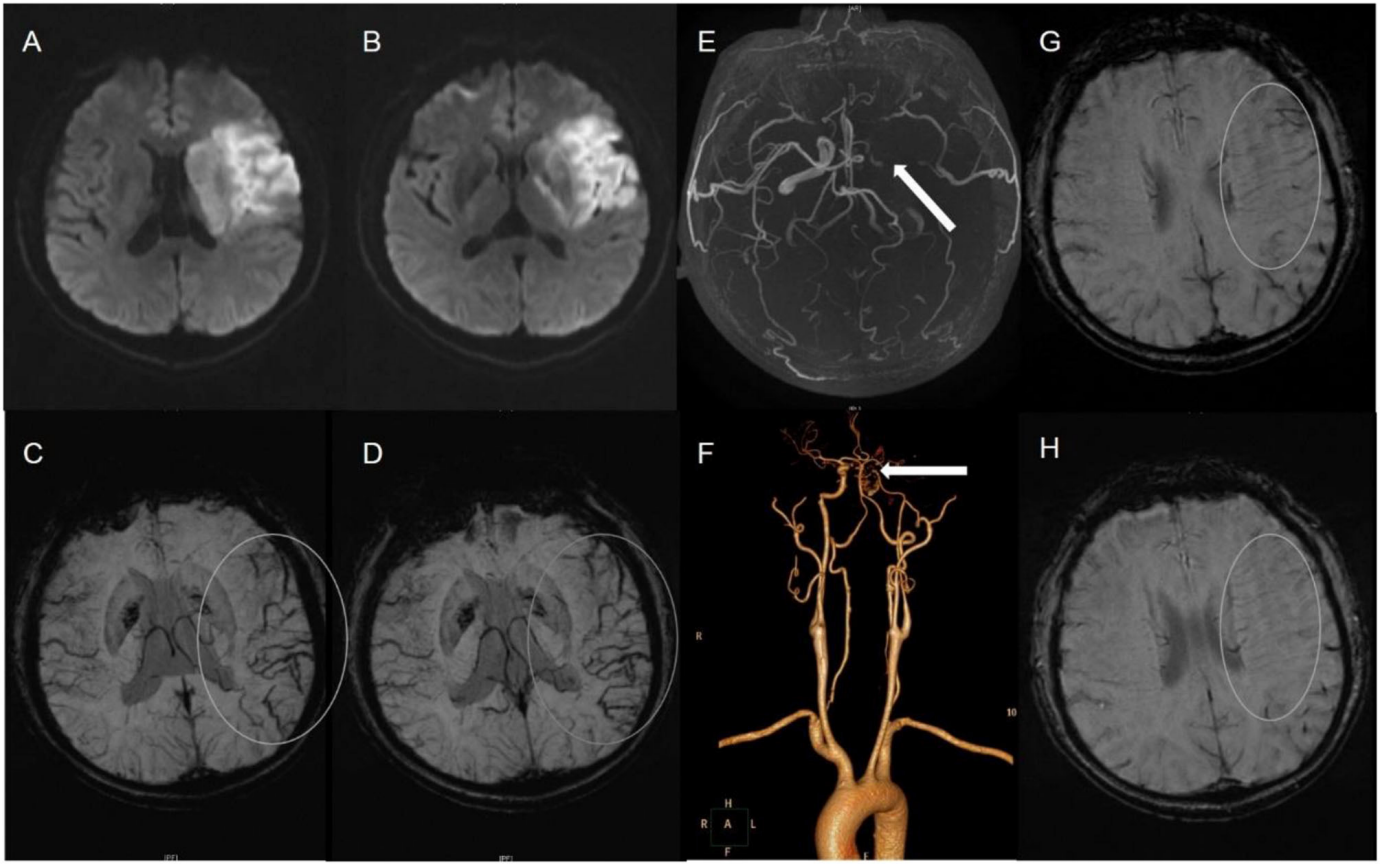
A 59-male patient with left TI (A,B). SWI indicates APCV in the left hemisphere [(C,D) white circle]. CTA showed left internal carotid artery occlusion [(F) white arrow]. The status of cerebral arterial circle was graded as poor with MCA was invisible [(E) white arrow]. The SWI showed prominent medullary veins hypointense signals (APMV) in the left hemisphere [(G,H) white circle].
